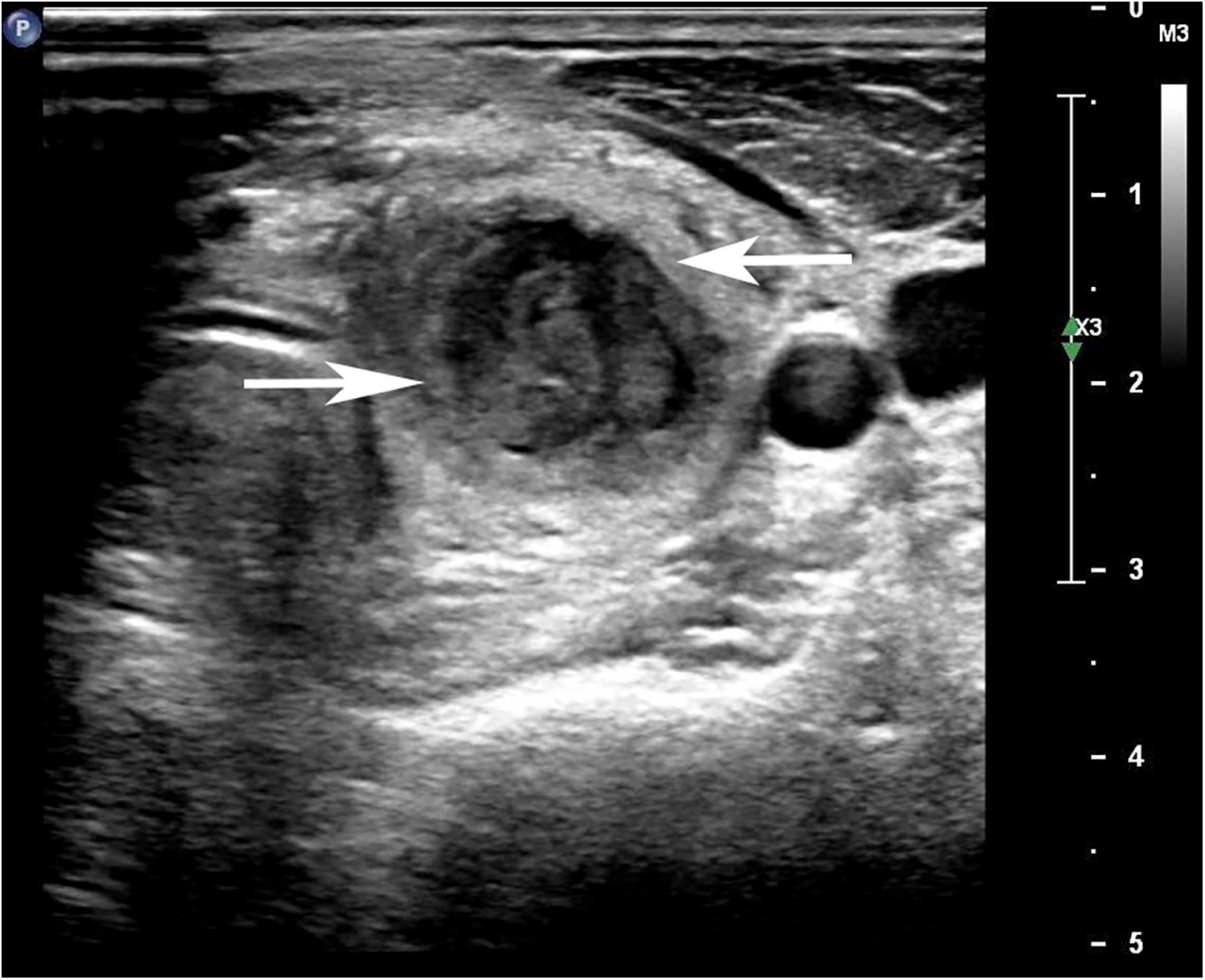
Ultrasound image of thyroid gland showing enlarged left lobe with ill-defined hypoechoic lesion with internal septations.
